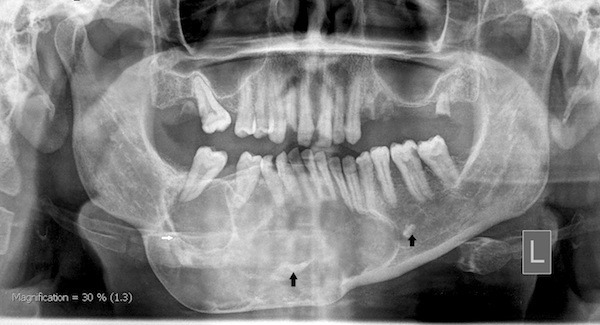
Panoramic radiograph showing an expansile multilocular lesion with radiopaque masses (arrows)
